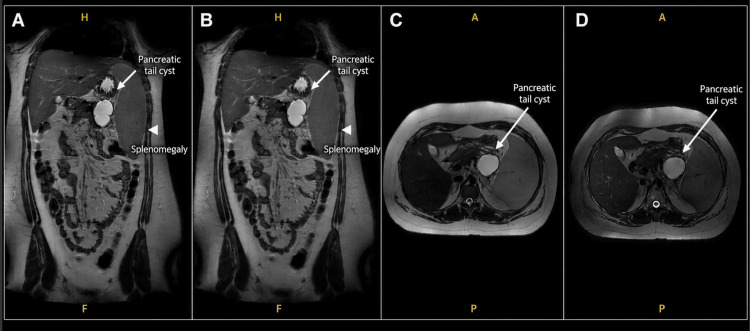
MRI abdomen demonstrating interval enlargement of the pancreatic tail cyst with associated splenomegaly. (A-B) Coronal T2-weighted images demonstrating a lobulated cystic lesion arising from the pancreatic tail (arrow) measuring approximately 5.6 × 4.6 × 4.9 cm. (C-D) Axial T2-weighted images confirming the pancreatic tail cyst (arrow). Associated splenomegaly is visible on both coronal and axial views. MRI: magnetic resonance imaging
